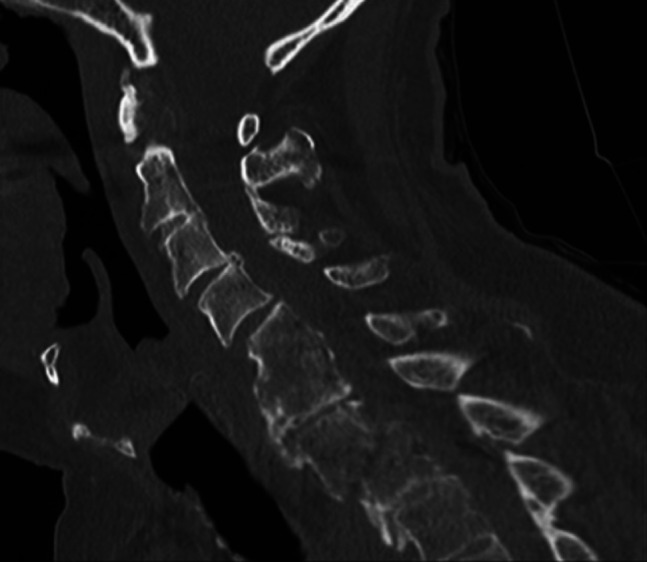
Representative preoperative sagittal CT cut demonstrating an oblique fracture through the C7-T1 ankylosed segment.
